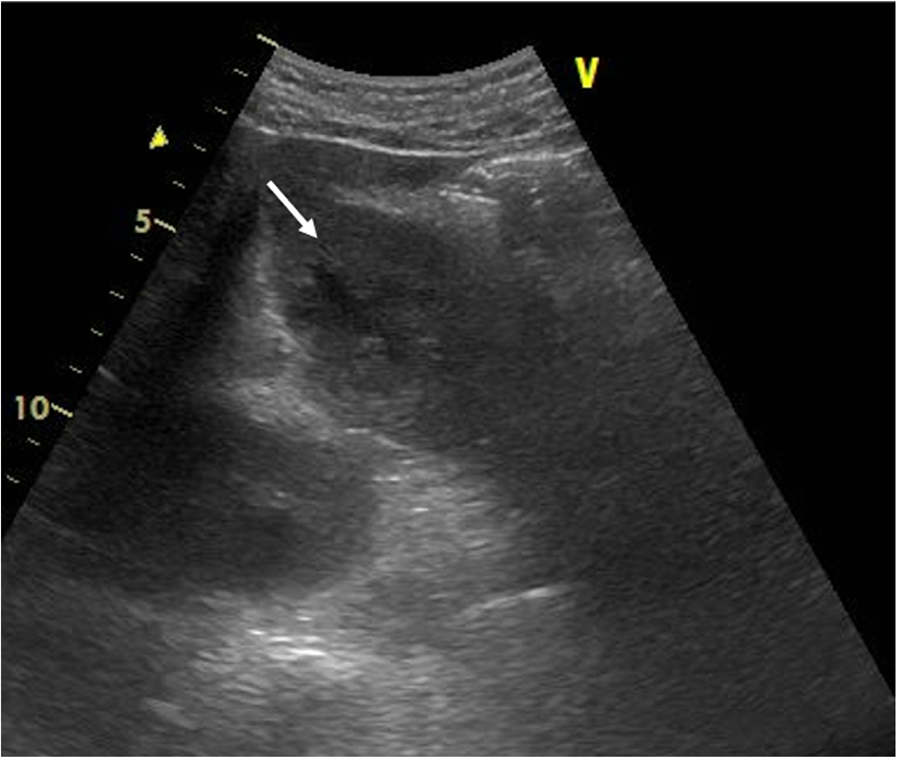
Abdominal ultrasonography findings. A hypoechoic lesion with an irregular margin (white arrow) was located in the left lobe of the liver
